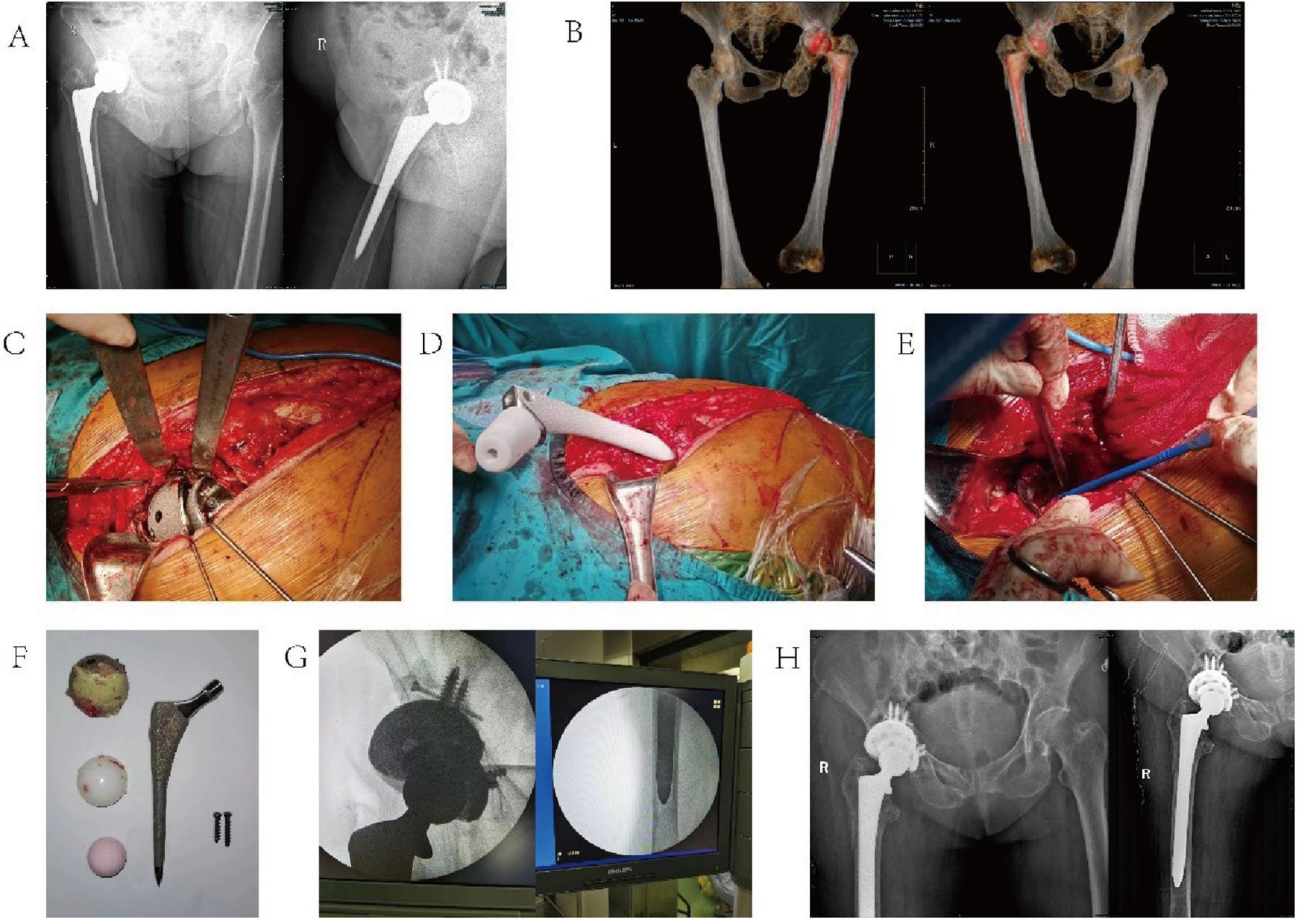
Typical case 1. (A) Preoperative hip X-ray; (B) preoperative CT; (C) intraoperative exposure of the acetabulum; (D) intraoperative placement of the bi-acetabular cup; (E) intraoperative installation of the femoral stem; (F) intraoperative removal of the prosthesis; (G) intraoperative fluoroscopy; and (H) postoperative hip X-ray.
